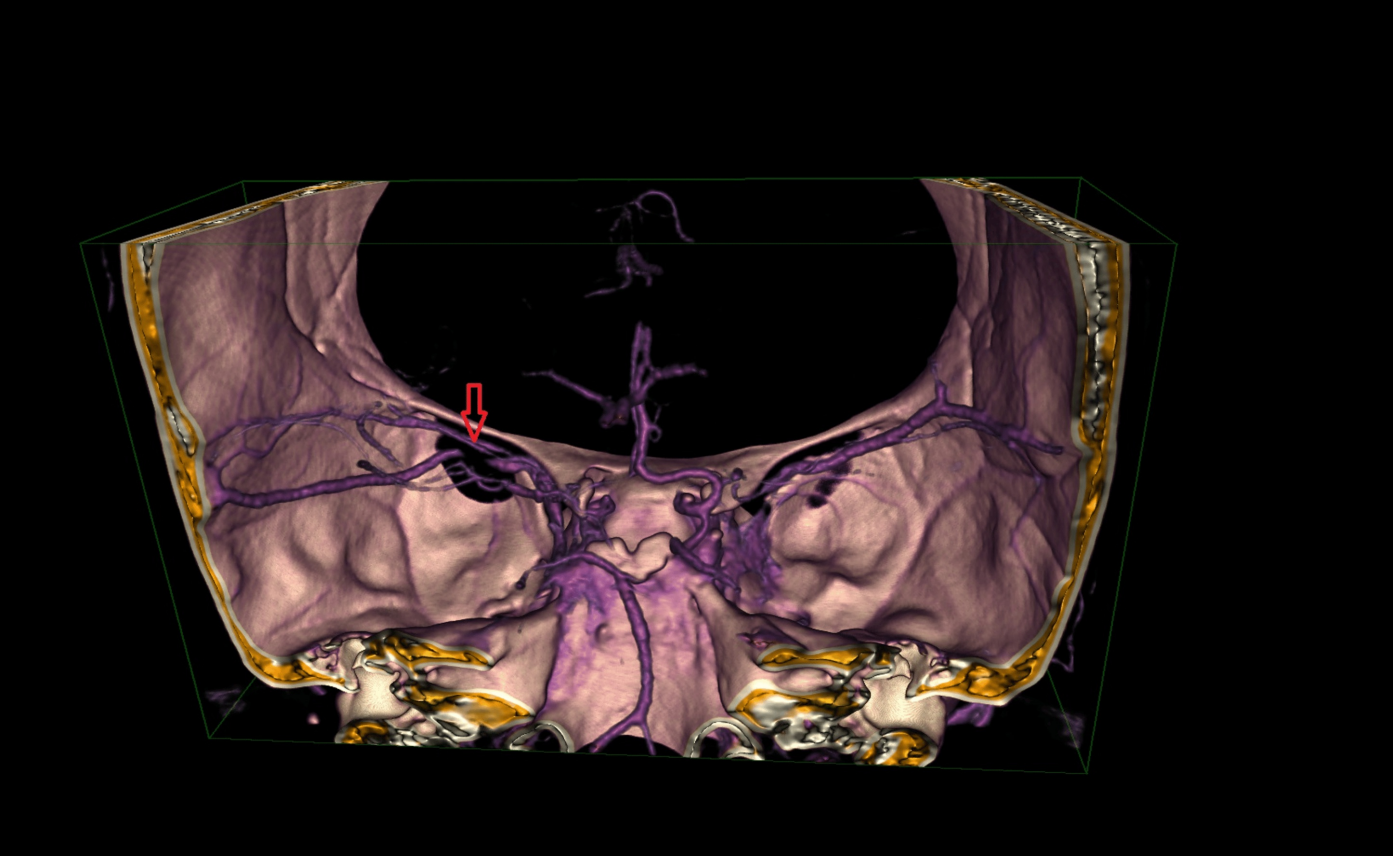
CT imaging in 3D demonstrating stenosis
